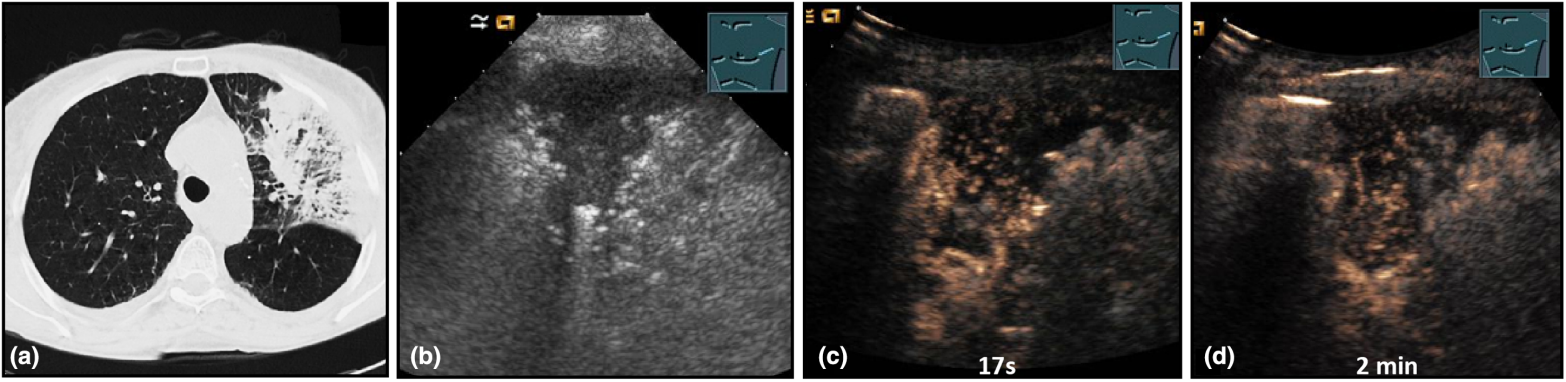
A 66‐year‐old patient presented with left‐sided bronchial carcinoma and shortness of breath and cough. The patient underwent percutaneous irradiation of the tumour region up to 50.4 Gy, with subsequent dose increase in the primary tumour area to 59.4 Gy. Computed tomography (courtesy of Prof. Dr. Andreas H. Mahnken, Department of Radiology, University Hospital Marburg) (a) shows a left‐sided peripheral lung infiltration; B‐mode ultrasound (b) shows a hypoechoic, irregularly bordered consolidation; contrast‐enhanced ultrasound reveals no pulmonary arterial perfusion. After 17 seconds (c) and after 2 minutes (d), a tender bronchial arterial perfusion can be detected, consistent with radiation pneumonitis.
